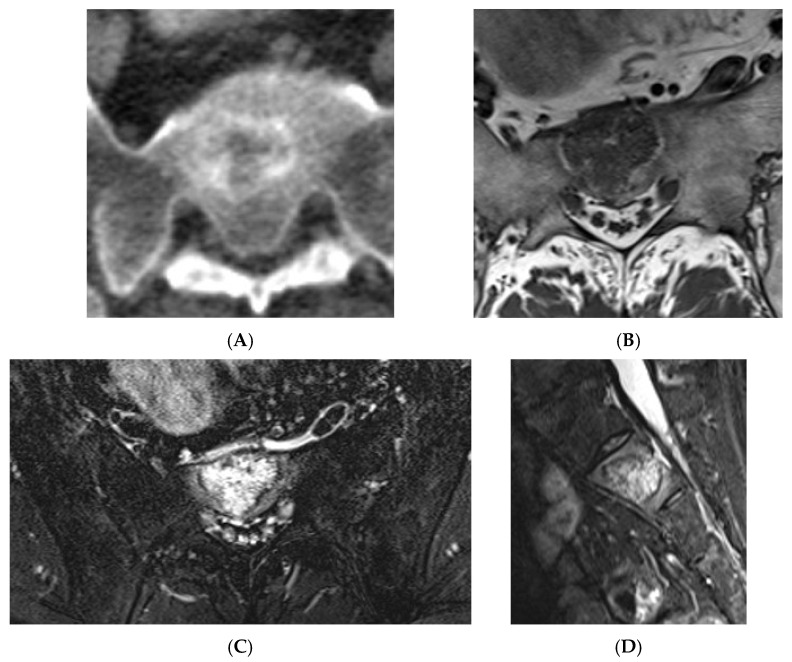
Atypical haemangioma at sacrum. (A) Axial CT. (B) T1W axial MRI. (C) Axial STIR MRI. (D) Sagittal STIR MRI.
